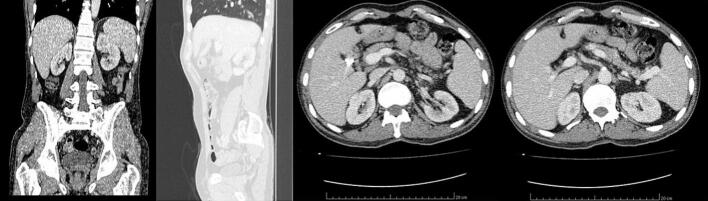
Different coronal slices of the post-operative abdominal CT scans showing no recurrence or metastases.
